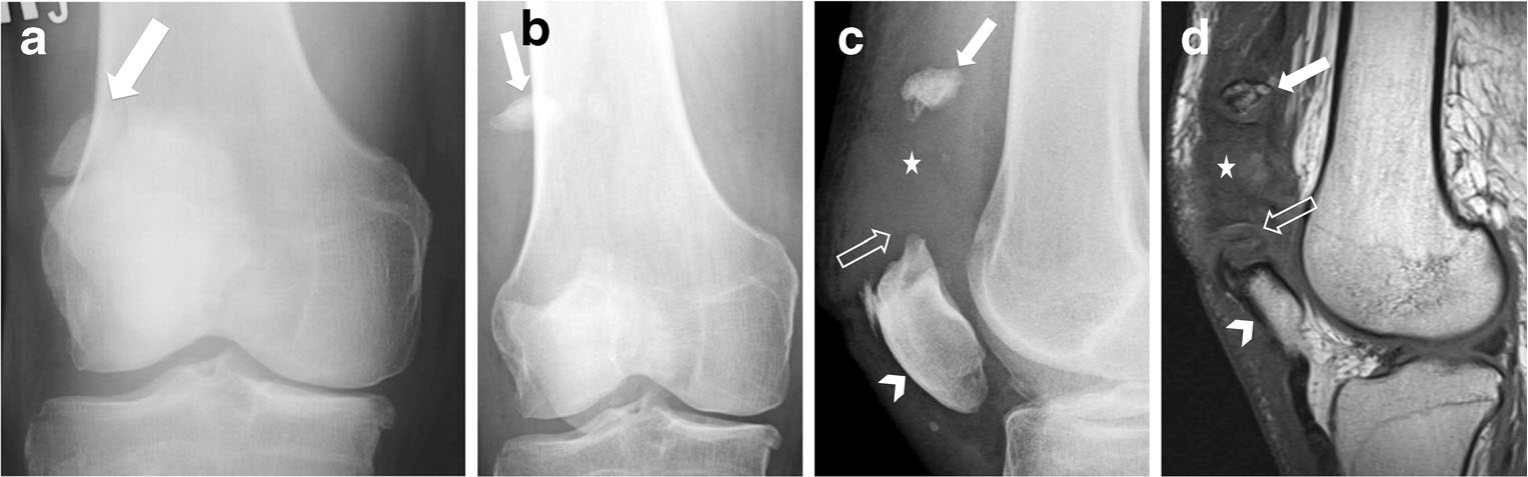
Separation of patella bipartite in a 70-year-old man. (a) Anteroposterior radiograph of the right knee demonstrates an incidental bipartite patella (*arrow*). Two years later, the patient presented with anterior knee pain after a fall. (b) Anteroposterior and (c) lateral radiographs and (d) sagittal T1-weighted MR image show separation of the superolateral fragment (*solid arrow*) within the anterolateral soft tissues of the knee, patella baja (*arrowhead*), obliteration of the suprapatellar fat (*open arrow*), and soft tissue swelling and haemorrhage from disruption of the quadriceps tendon (*star*)
